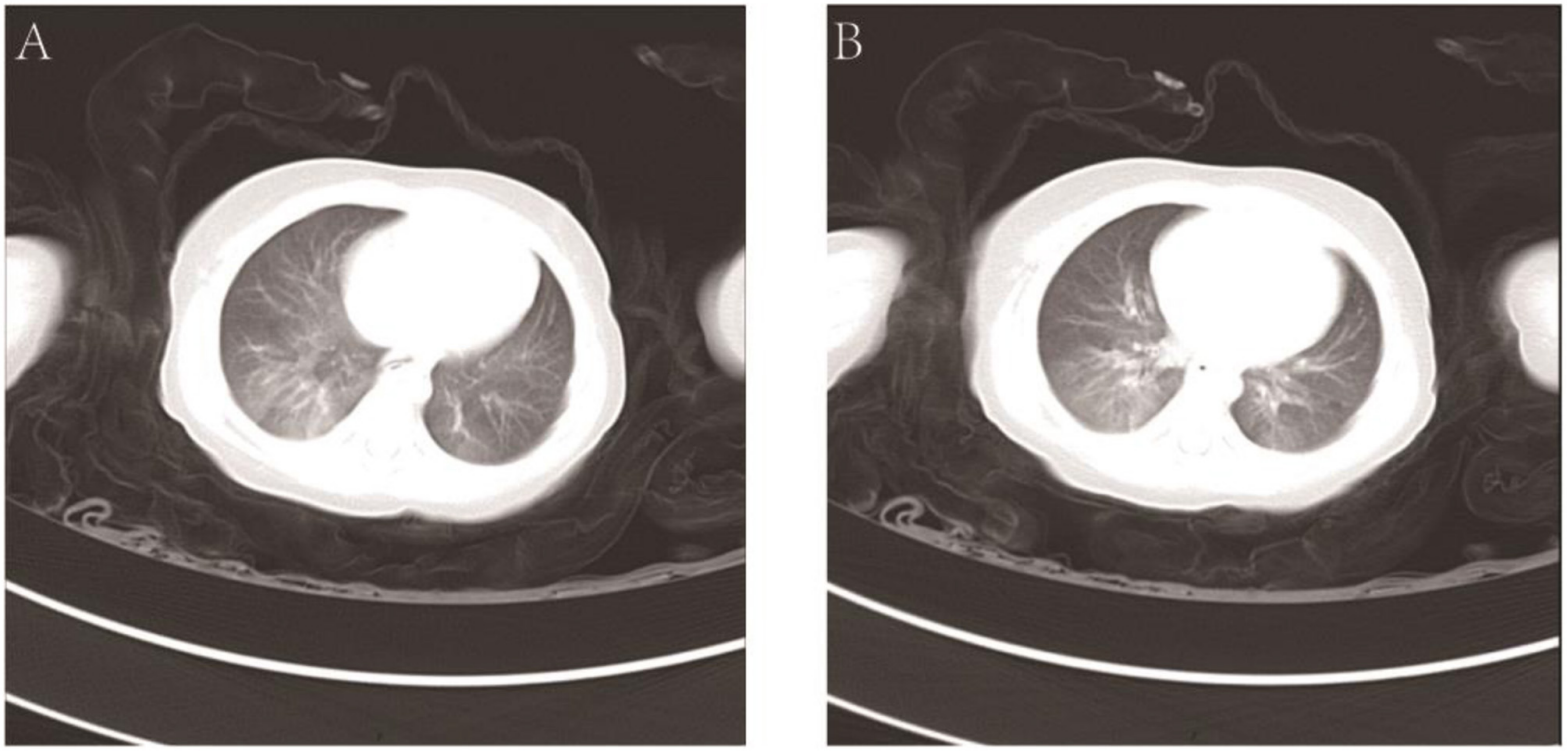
CT coronal scan of the lung (A,B).
